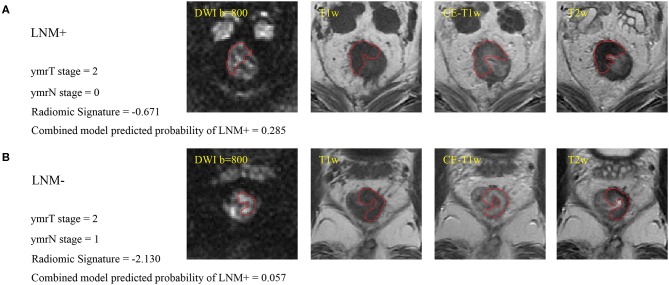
(A) Sample images of LNM+, where the red line indicates the tumor margin. (B) Sample images of LNM–. Cutoffs for the radiomic signature and combined model are −1.4208 and 0.0897, respectively, for patients with ymrT1-2 tumors in this study. These two patients were misdiagnosed by the radiologist but were correctly assessed by radiomics analysis. LNM+, lymph node metastasis; LNM–, lymph node non-metastasis; DWI, diffusion-weighted imaging; T1w, T1-weighted; CE-T1w, contrast-enhanced T1-weighted; T2w, T2-weighted.
